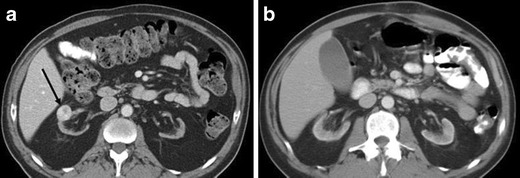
A 58-year-old man with a right renal oncocytoma arising from end-stage renal disease. a The portal venous phase of the axial CE-CT depicts a well-circumscribed enhancing mass (*arrow*) in the mid portion of the right kidney. Note that the bilateral kidneys are atrophic. b CE-CT 9 years prior demonstrates no evidence of a renal mass
